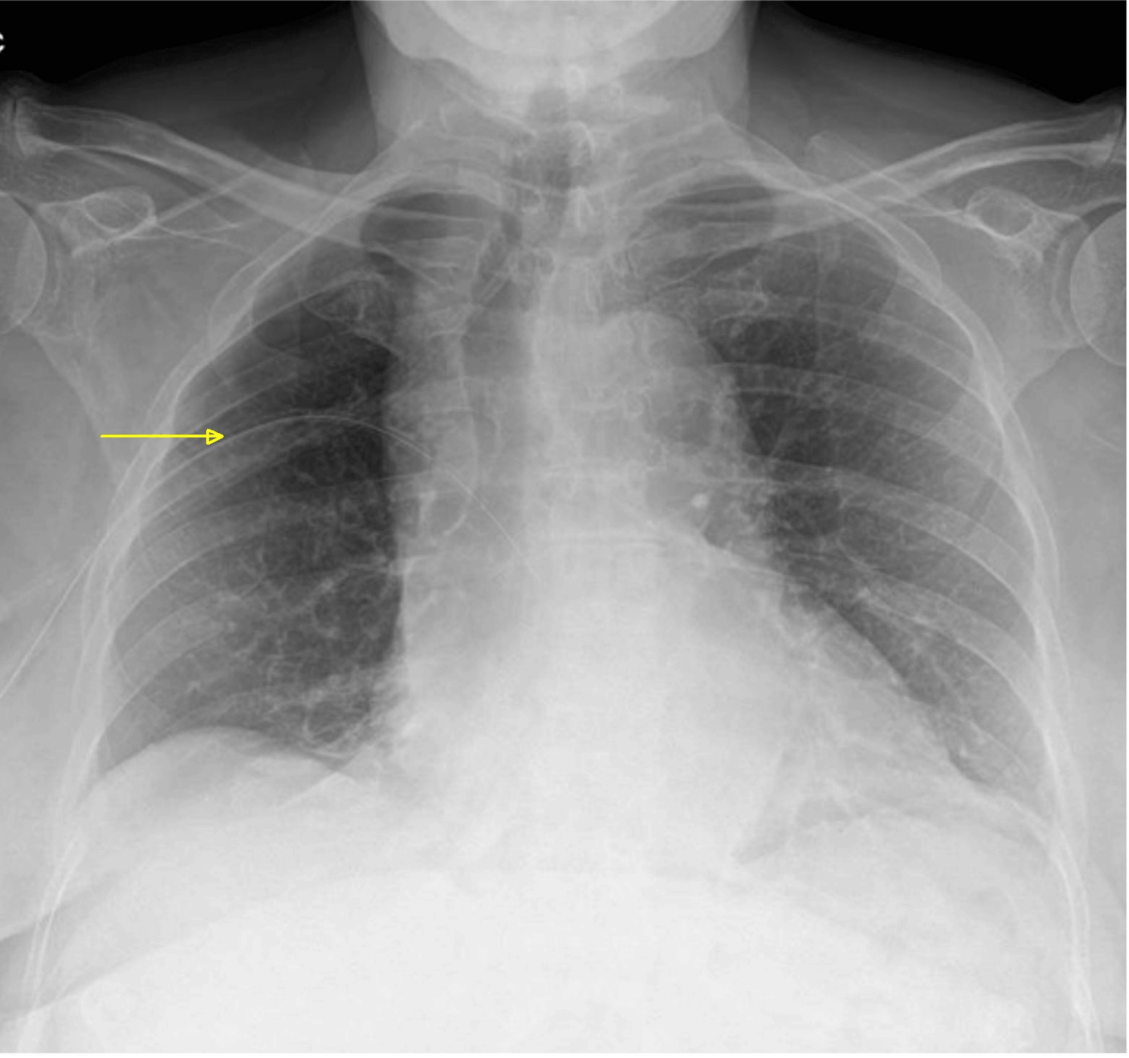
Posteroanterior erect chest X-ray 24 hours following the insertion of chest drain The arrow points to the drain position
